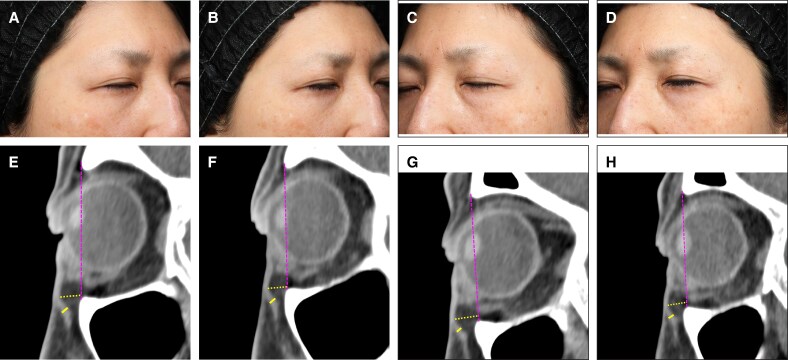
Photographs of the control side at (A) baseline and (B) week 8 in a 54-year-old female participant, showing no perceptible change in the infraorbital region. Photographs of the neuromuscular electrical stimulation (NMES)-applied side (C) at baseline and (D) week 8, showing a visible reduction in the baggy eyelid and attenuation of the nasojugal groove after treatment. (E, F) Sagittal computed tomography (CT) images of the control side at baseline and week 8, respectively. Orbicularis oculi muscle (OOM) thickness (thick yellow line) remained unchanged at 1.47 mm, and the length of orbital fat herniation (thin yellow line) decreased slightly from 5.53 to 5.50 mm (−0.60%). (G, H) Sagittal CT images of the NMES-applied side at baseline and week 8, respectively. OOM thickness increased from 1.40 to 1.60 mm (14.29%), and the length of orbital fat herniation decreased from 6.33 to 5.83 mm (−7.89%).
